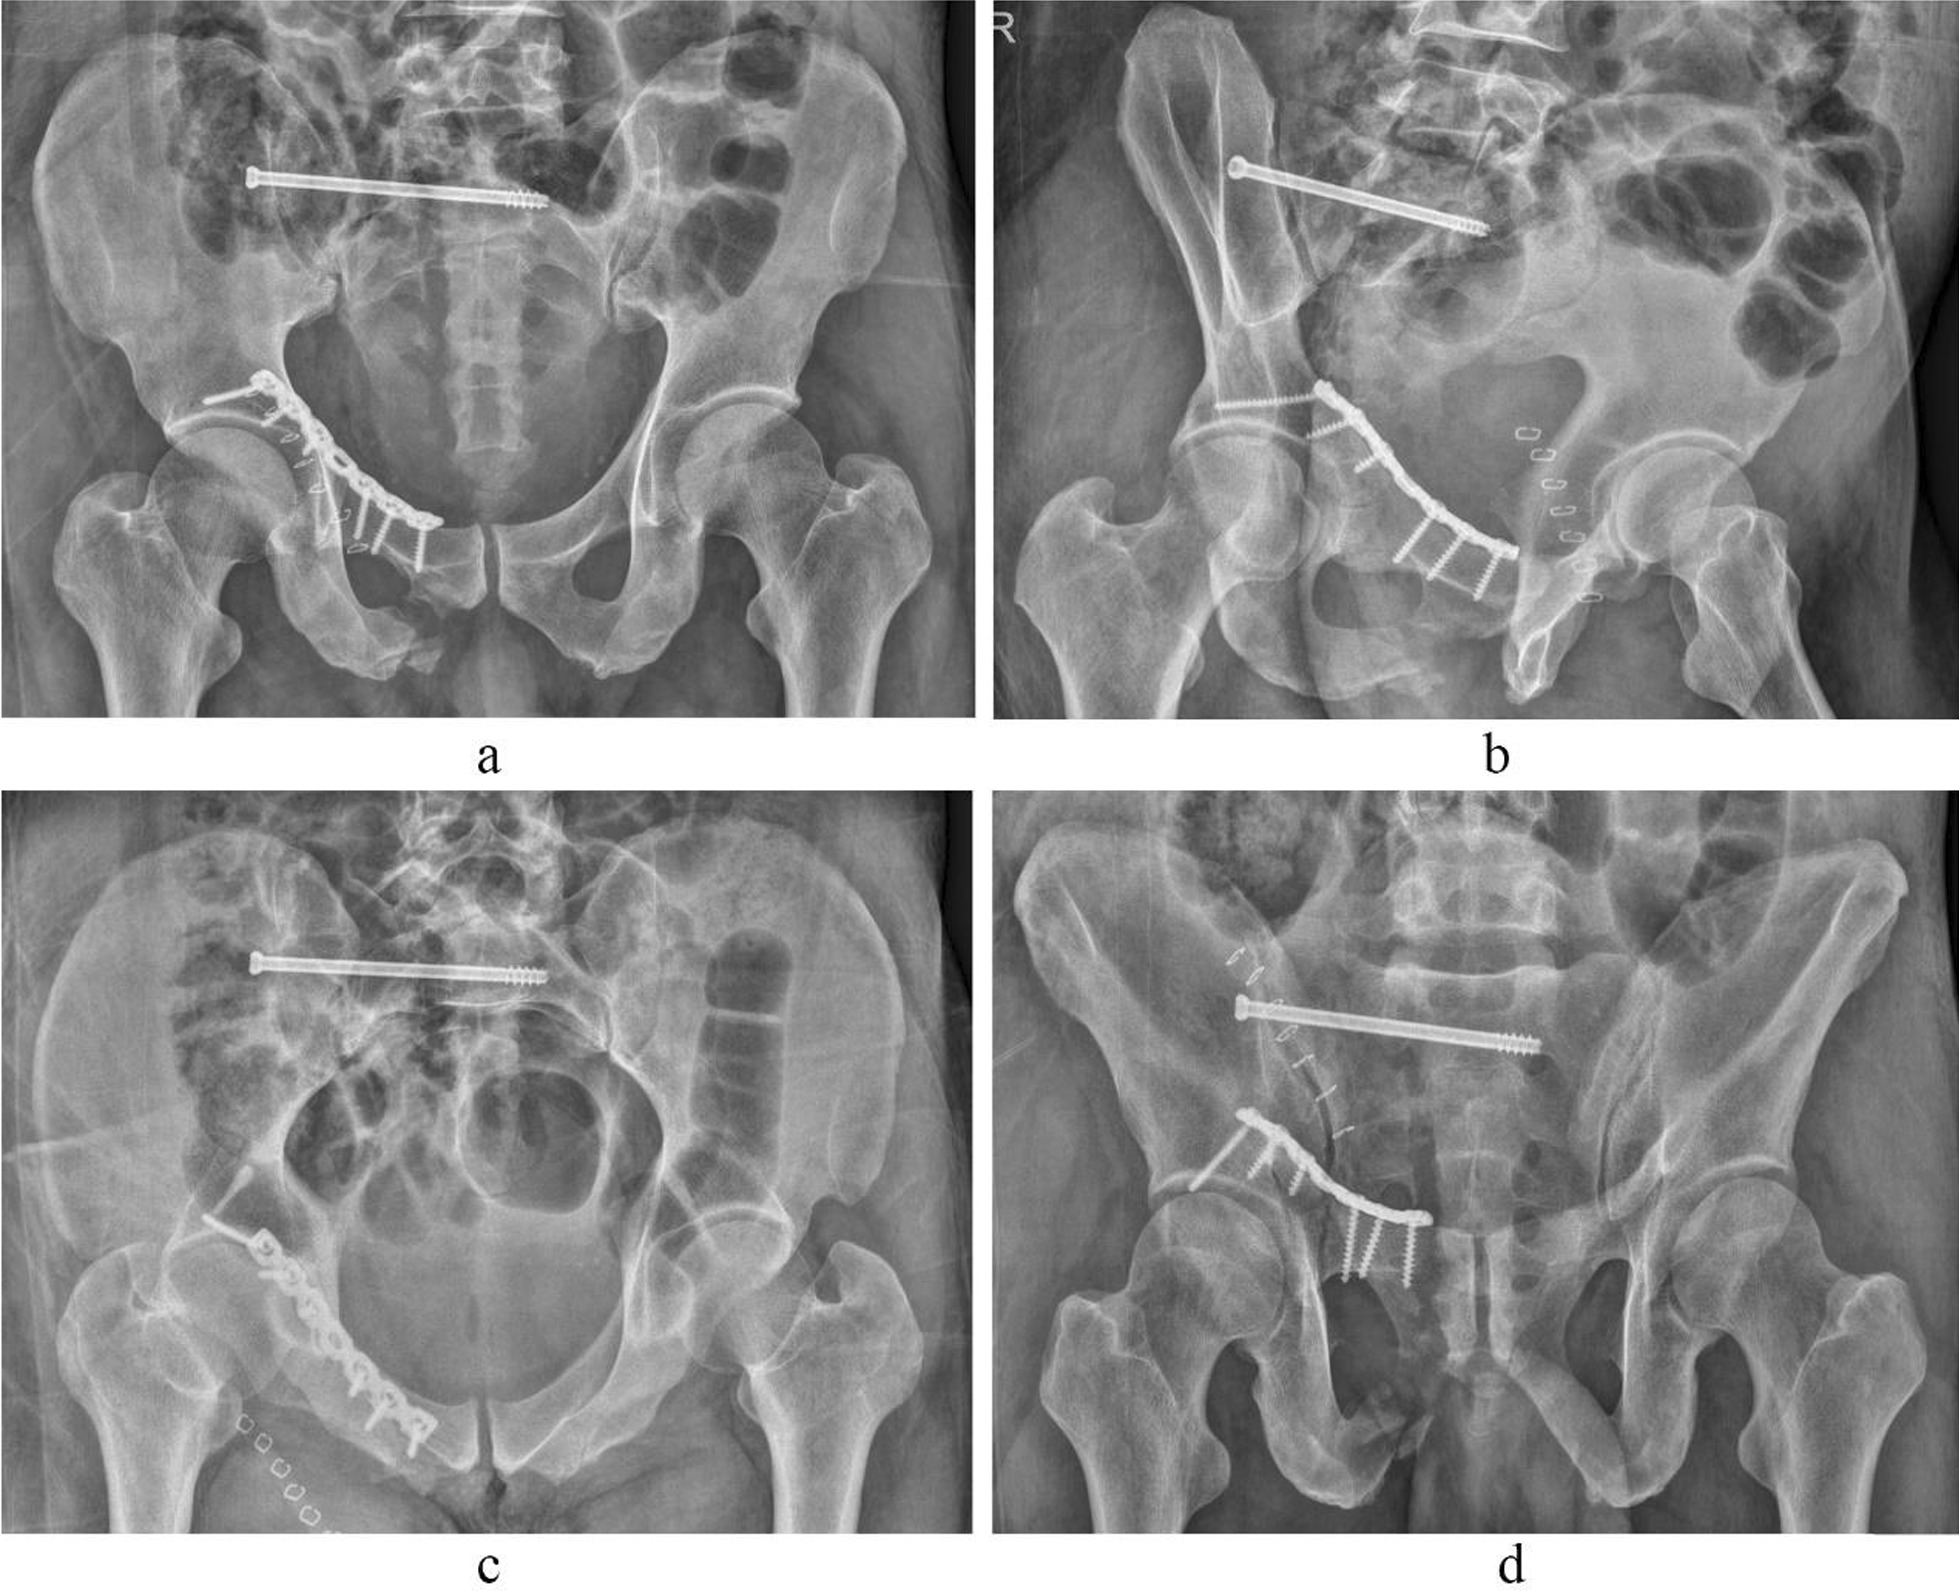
Postoperative X-ray images of case 4. (a Pelvic anteroposterior X-ray image, b obturator oblique position X-ray image, c pelvic inlet X-ray image, d pelvic outlet X-ray image.)
